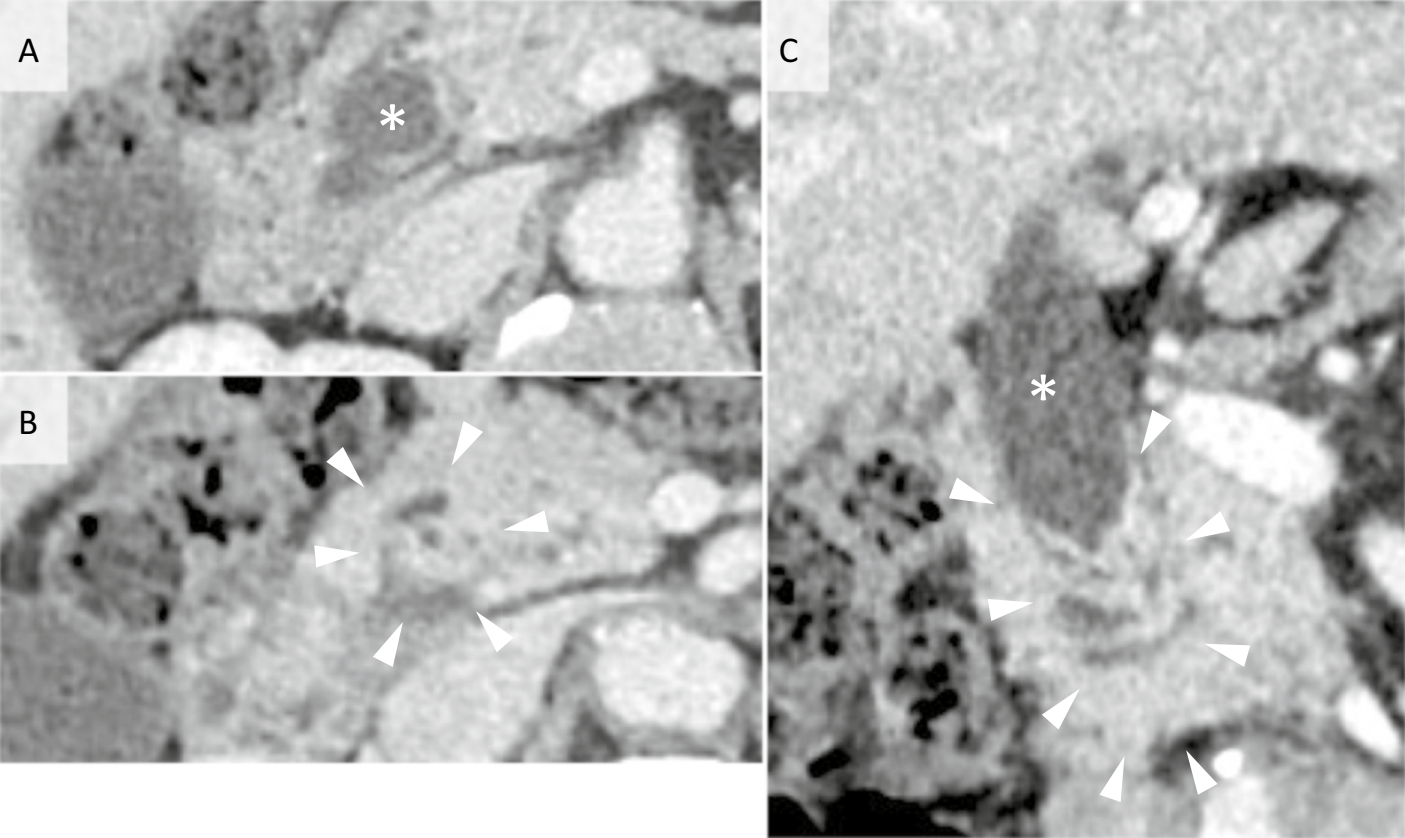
The preoperative contrast-enhanced CT scan. A Axial view showing dilated proximal side of common bile duct (asterisk). B An irregular mass lesion in middle bile duct (arrowheads). C Coronal view showing dilated proximal bile duct (asterisk) and multiple overlapping leaf-like folds in the middle bile duct (arrowheads)
